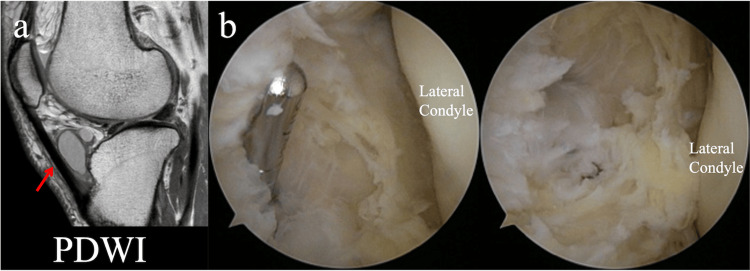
Images of Case 2 (a) Magnetic resonance image of the knee. As in case 1, a multifocal mass was found in the infrapatellar fat pad, (b) Arthroscopic views. As in case 1, the mass wall was excised until the normal sublabial fat pad was exposed.
